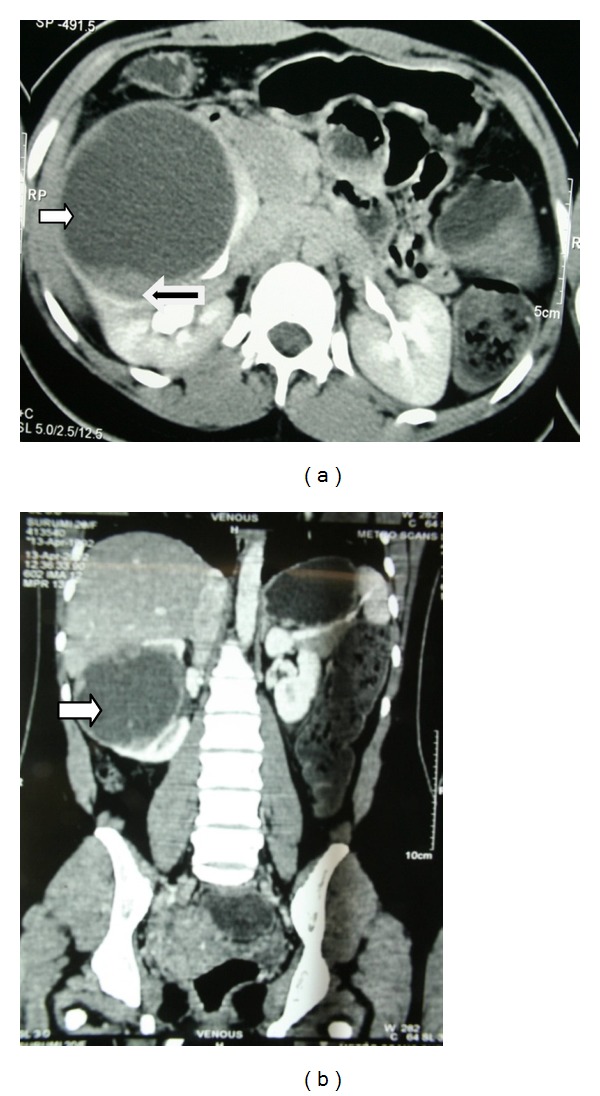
Contrast enhanced CT scan of the abdomen and pelvis in patient 1 with (a) axial section and (b) coronal section showing a large predominantly cystic (white arrow) right renal mass measuring 9 × 8 × 8 cm with solid areas (black arrow).
